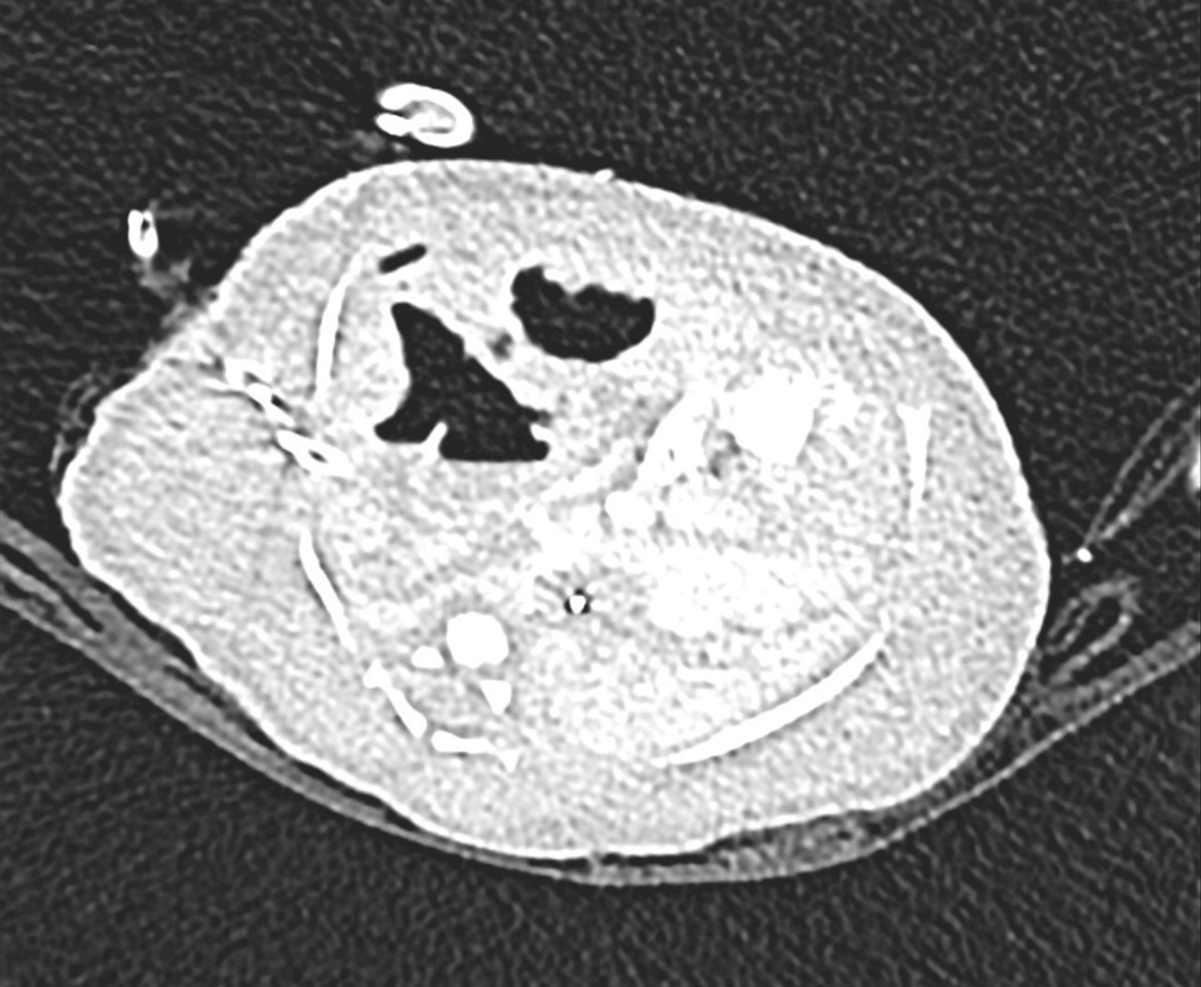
Chest CT of the newborn showing a right middle lobe CPAM A large right middle lobe congenital cystic malformation occupies much of the right hemithorax, with a significant contralateral mediastinal shift. A relatively small patent ductus arteriosus is also noted. CPAM, congenital pulmonary airway malformation
